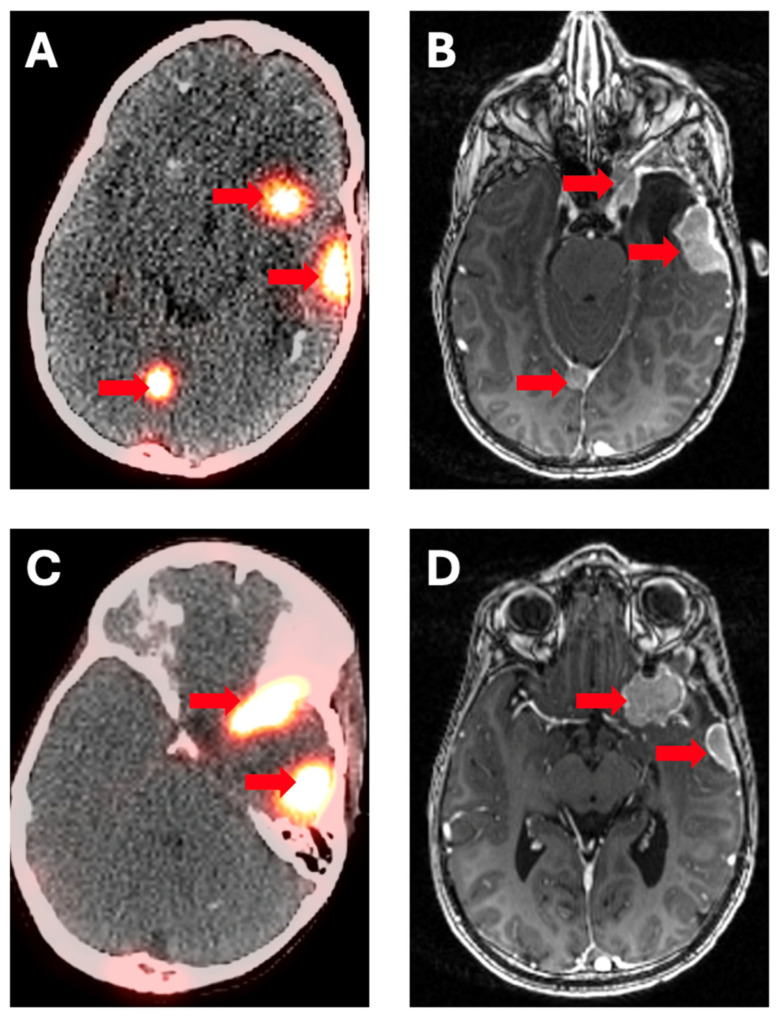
Images from a 48-year-old woman with multi-focal meningiomas. (A,C) Axial ^68^Ga-DOTATATE PET/CT images demonstrate tense uptake at multiple intracranial sites (arrows) that correspond to enhancing mass lesions on (B,D) contrast-enhanced, post-contrast, T1-weighted MRI (arrows).
